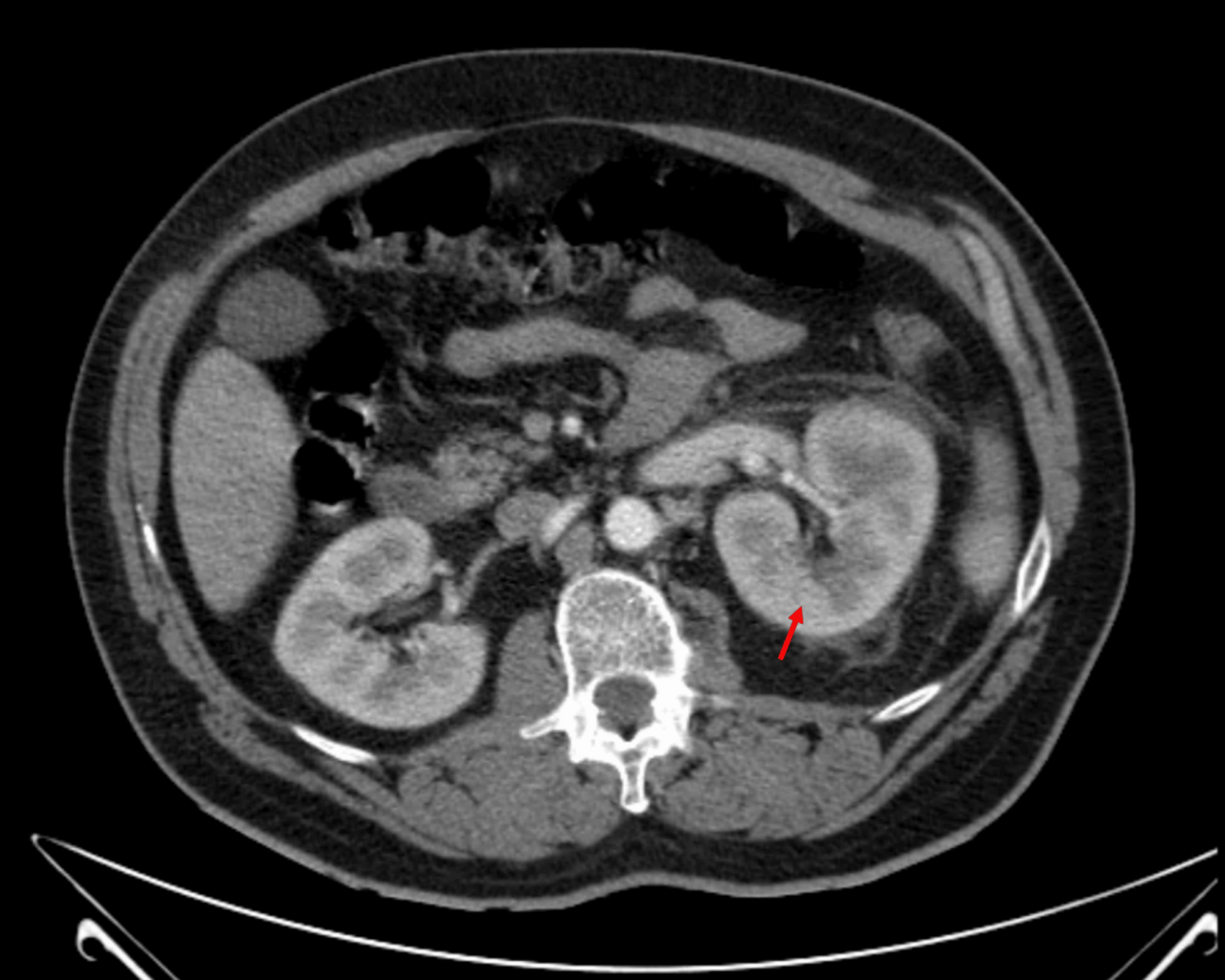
Contrast-enhanced abdominal CT scan shows hypodense area (red arrow), suggestive of focal pyelonephritis and no sign of subcapsular hematoma or other complications.
